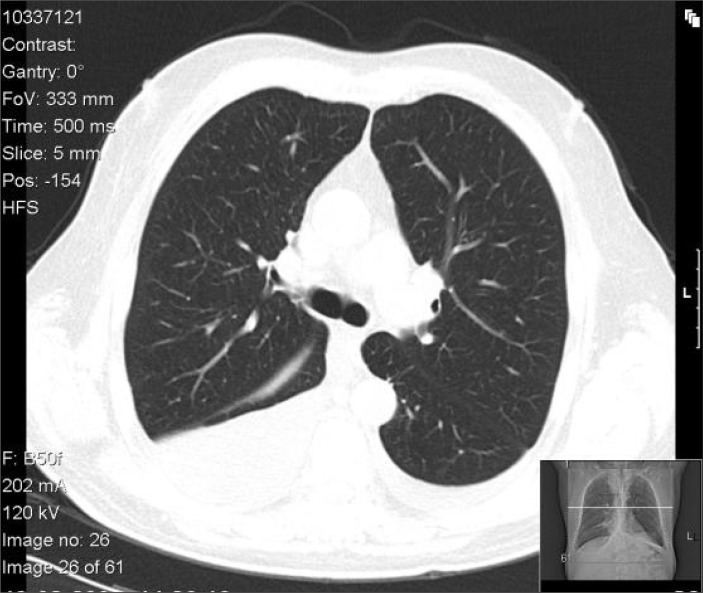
Features of computed tomography of the chest.
